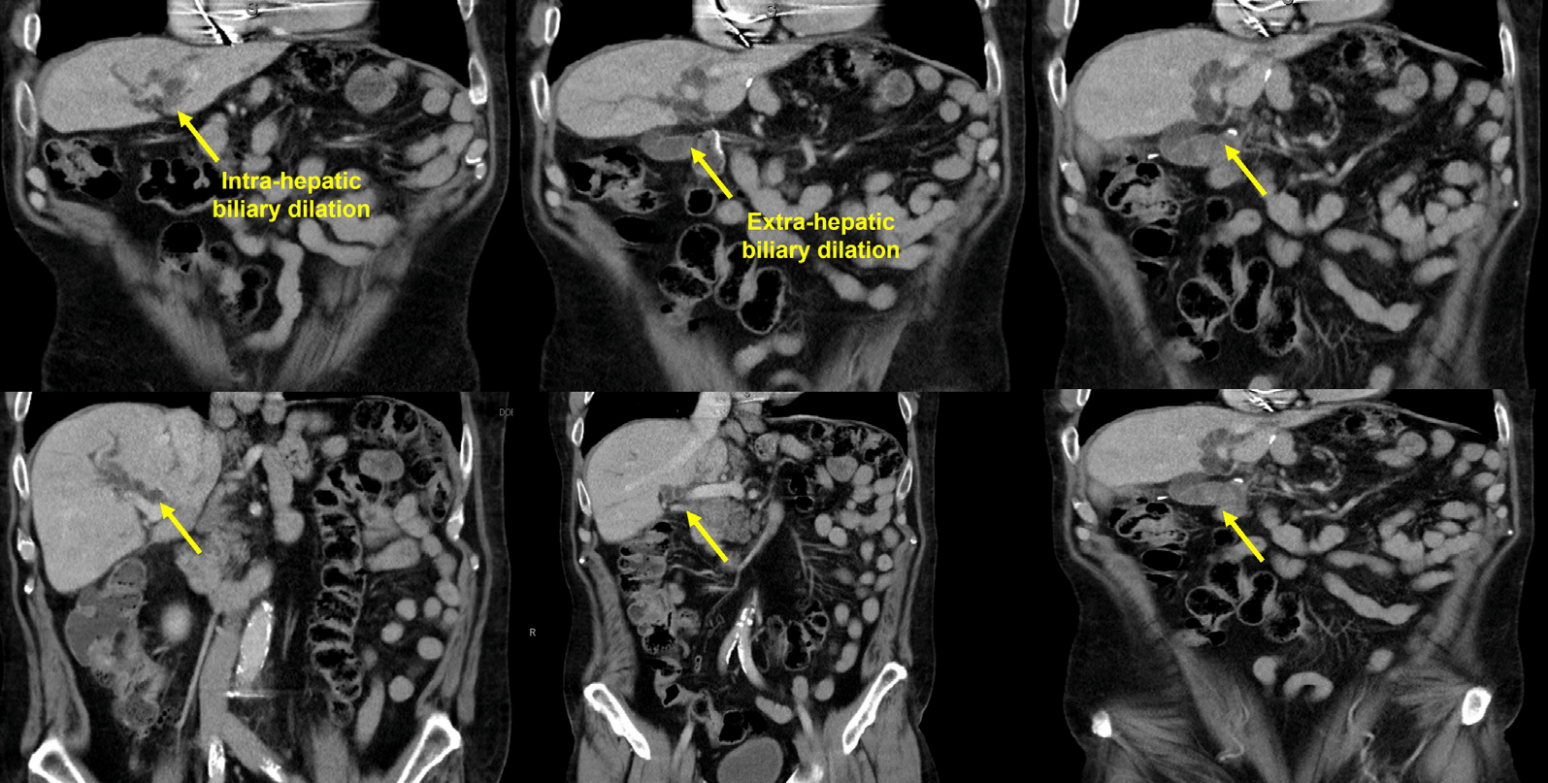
Coronal plane liver CT scan indicating dilation of the intrahepatic and extrahepatic bile ducts.
